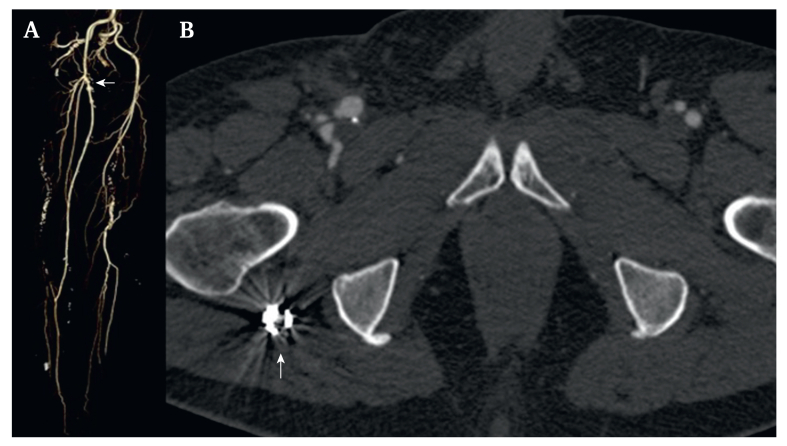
(A) Coronal volume rendering technique and (B) axial computed tomographic images demonstrated the successful exclusion of the persistent sciatic artery aneurysm and patency of the bypass between the common femoral artery (arrow) and the right posterior tibial artery.
